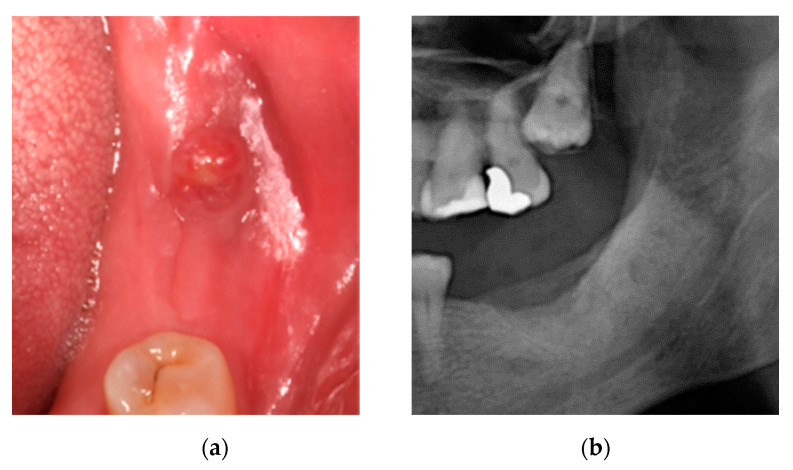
(a) Fistula in the jaw and (b) X-ray of the affected area.
